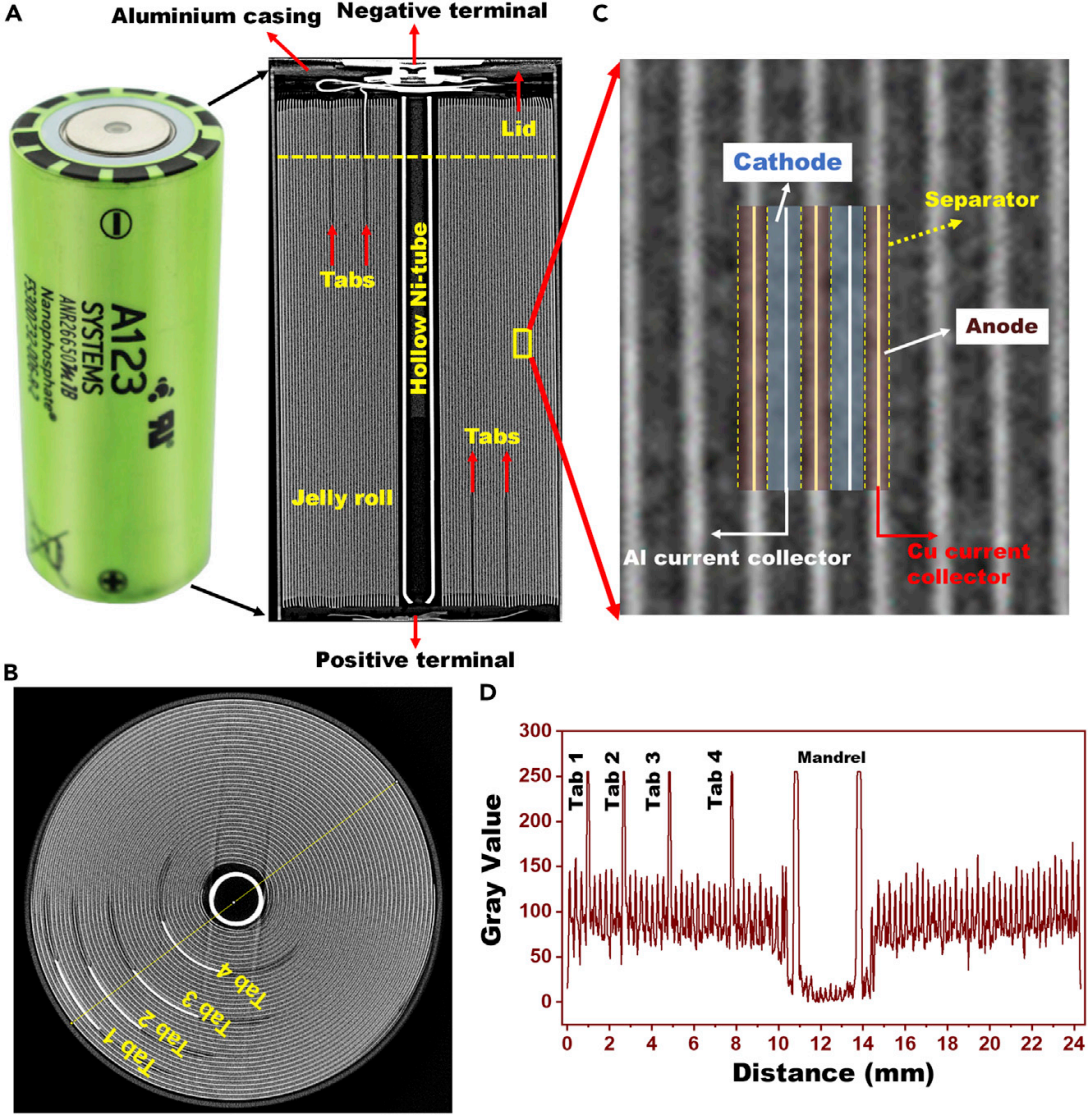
CT scan images of 26650 cylindrical commercial LiFePO_4_/graphite lithium-ion cell (A) the pristine cell and its vertical cross-section image, (B) electrode jelly roll, (C) magnified view of the electrodes and separators in the jelly roll, and (D) line profile along the diameter of the jelly roll in (B).
